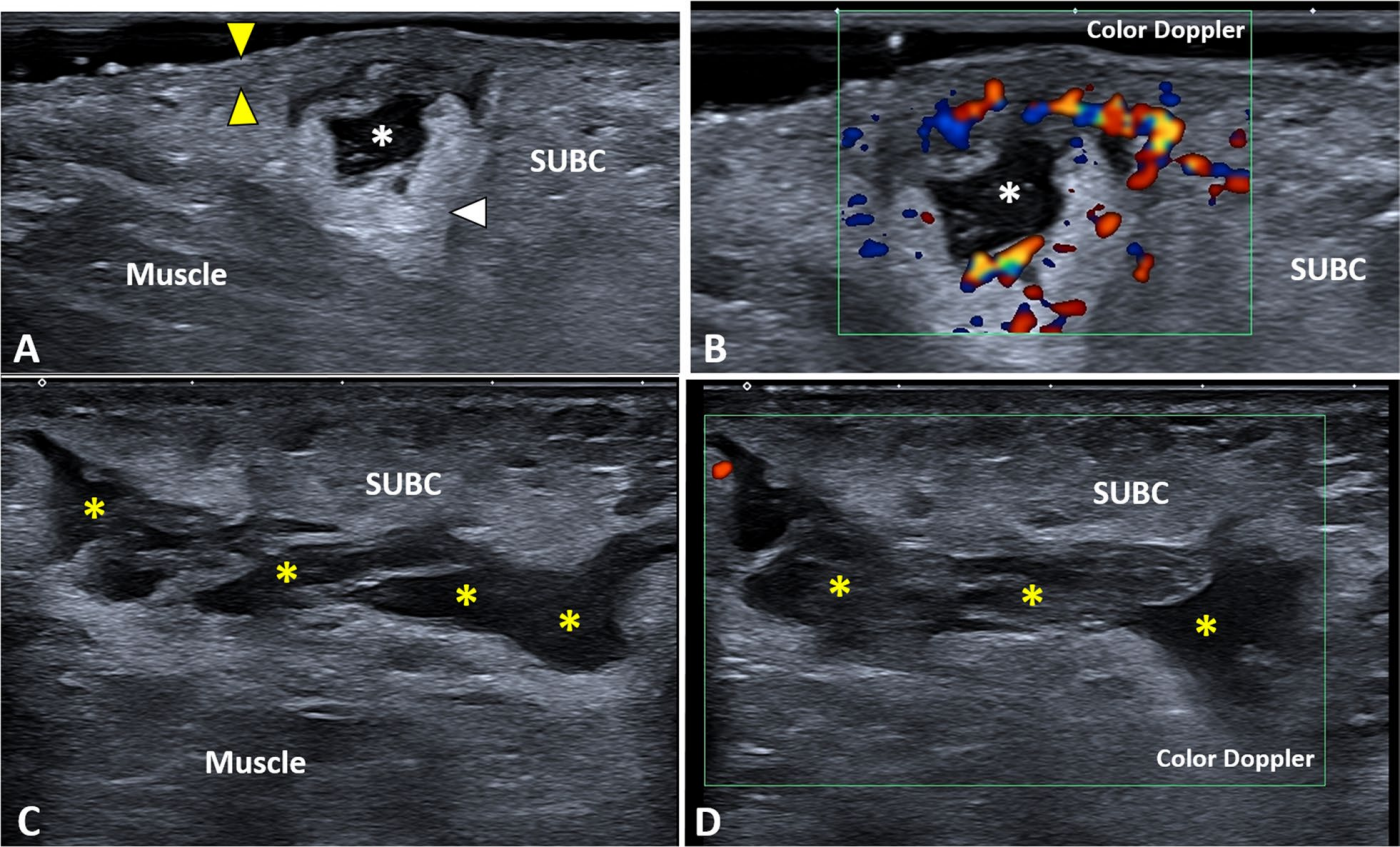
Subcutaneous abscess vs. hematoma of the axilla. A small, irregular-in-shape, fluid collection *(white asterisk)* can be identified within the axillary subcutaneous tissue *(SUBC)* not extending to the overlying dermo-epidermal complex *(yellow arrowheads)* nor to the underlying muscle planes (A); and high-sensitivity color Doppler shows intense hypervascularization of the surrounding soft tissues confirming inflammatory/infectious local phenomena (B). Of note, axillary hematoma *(yellow asterisks)* can present a sonographic pattern mimicking an abscess (C), but vascular signals are usually absent within the surrounding fat pad (D). White arrowhead: posterior acoustic enhancement
